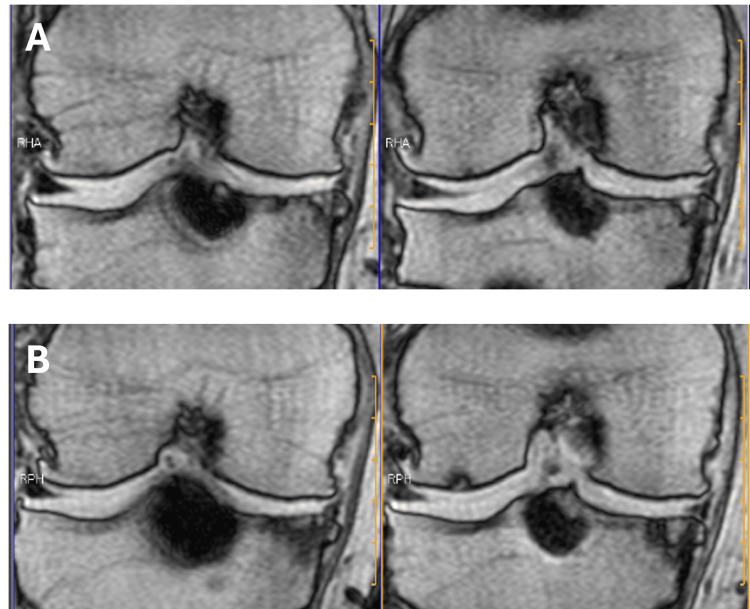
MRI showed that the intercondylar notch was well formed after arthroscopic surgery: (A) before arthroscopic surgery and (B) one year after arthroscopic surgery. MRI: magnetic resonance imaging
